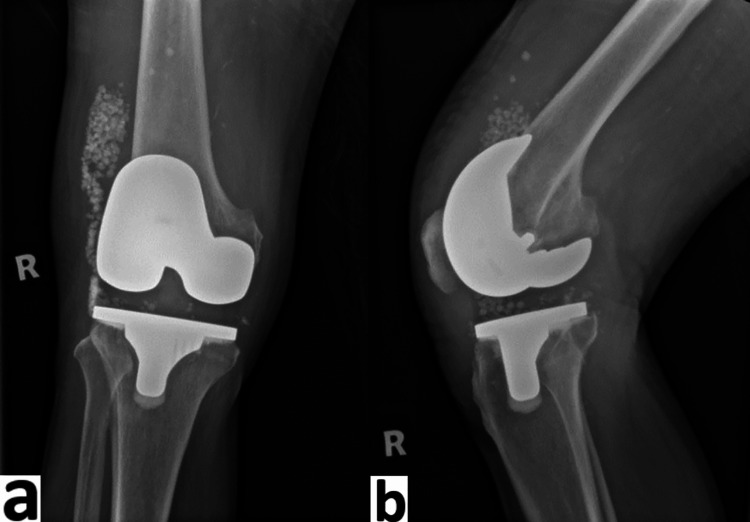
Radiograph of right knee postoperatively a) anteroposterior view; b) lateral view
